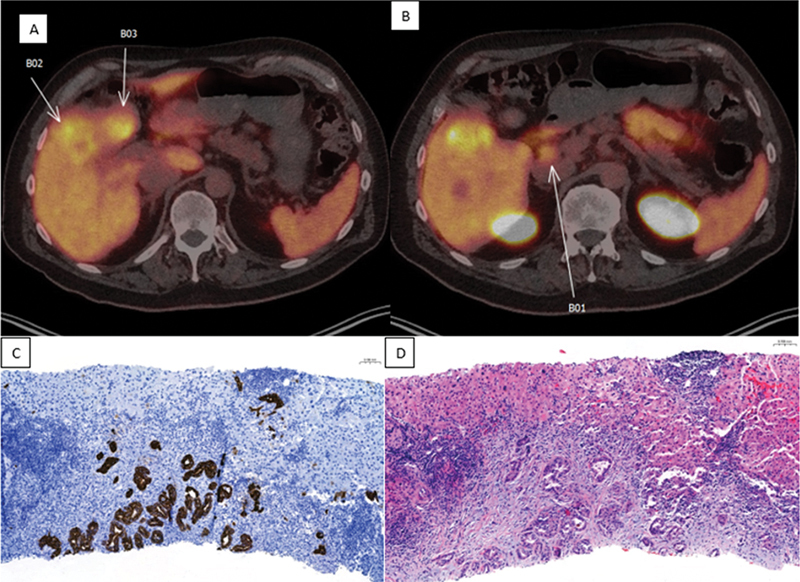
Fluorine-18 (F-18) PYL prostate-specific membrane antigen positron emission tomography/computed tomography cross-sectional image showing (
